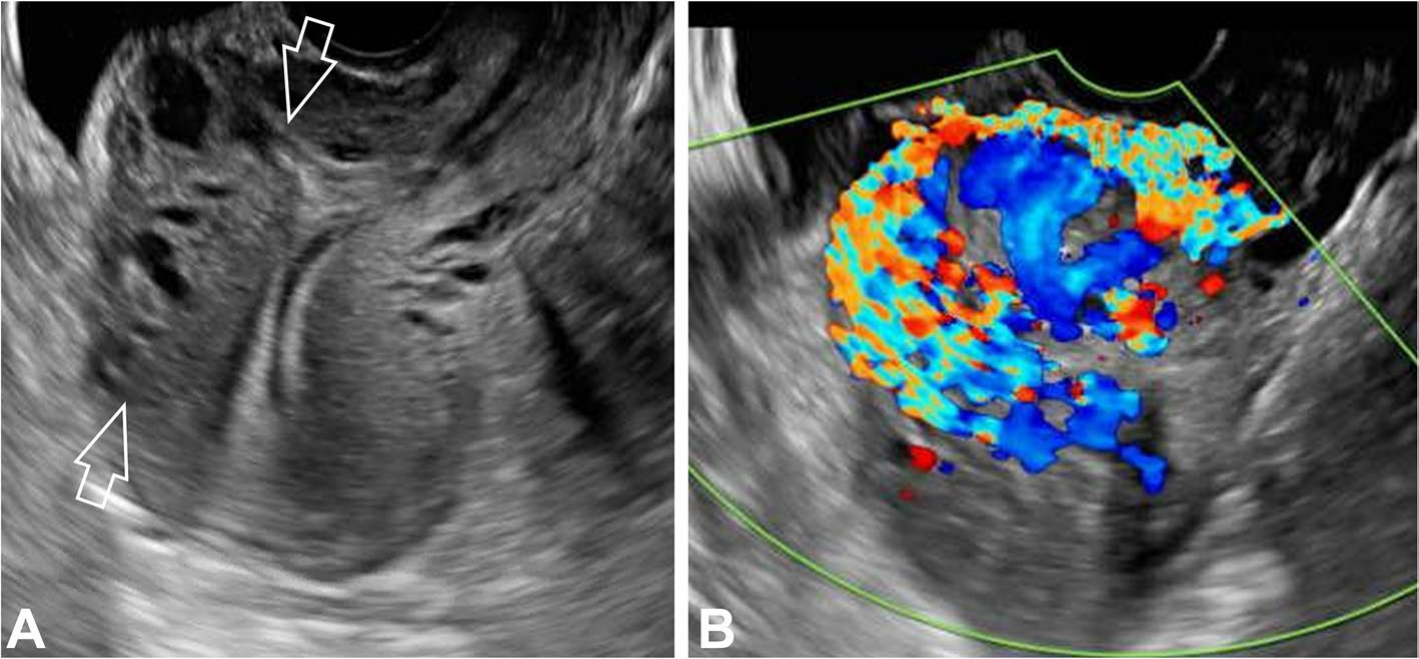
Transvaginal Doppler US of RPOC observed as a heterogeneous endocavitary mass (open arrows in A) with marked vascularity on Doppler-US (image B)
